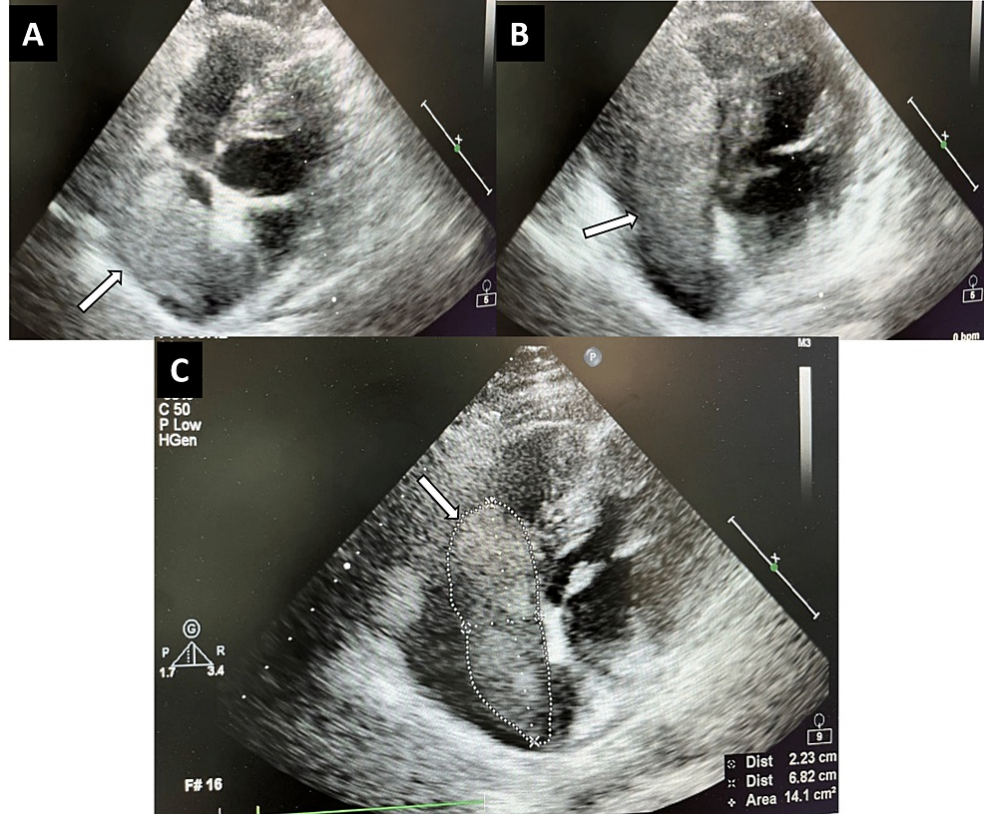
Transthoracic echocardiography showing a huge mass in the right atrium. (A) A rather large, highly mobile mass (white arrows in A,B) in the right atrium (B) moves towards the right ventricle with cardiac contractions. (C) Total area of the mass (white arrow/circled mass in C) is 14.1 cm^2^.
